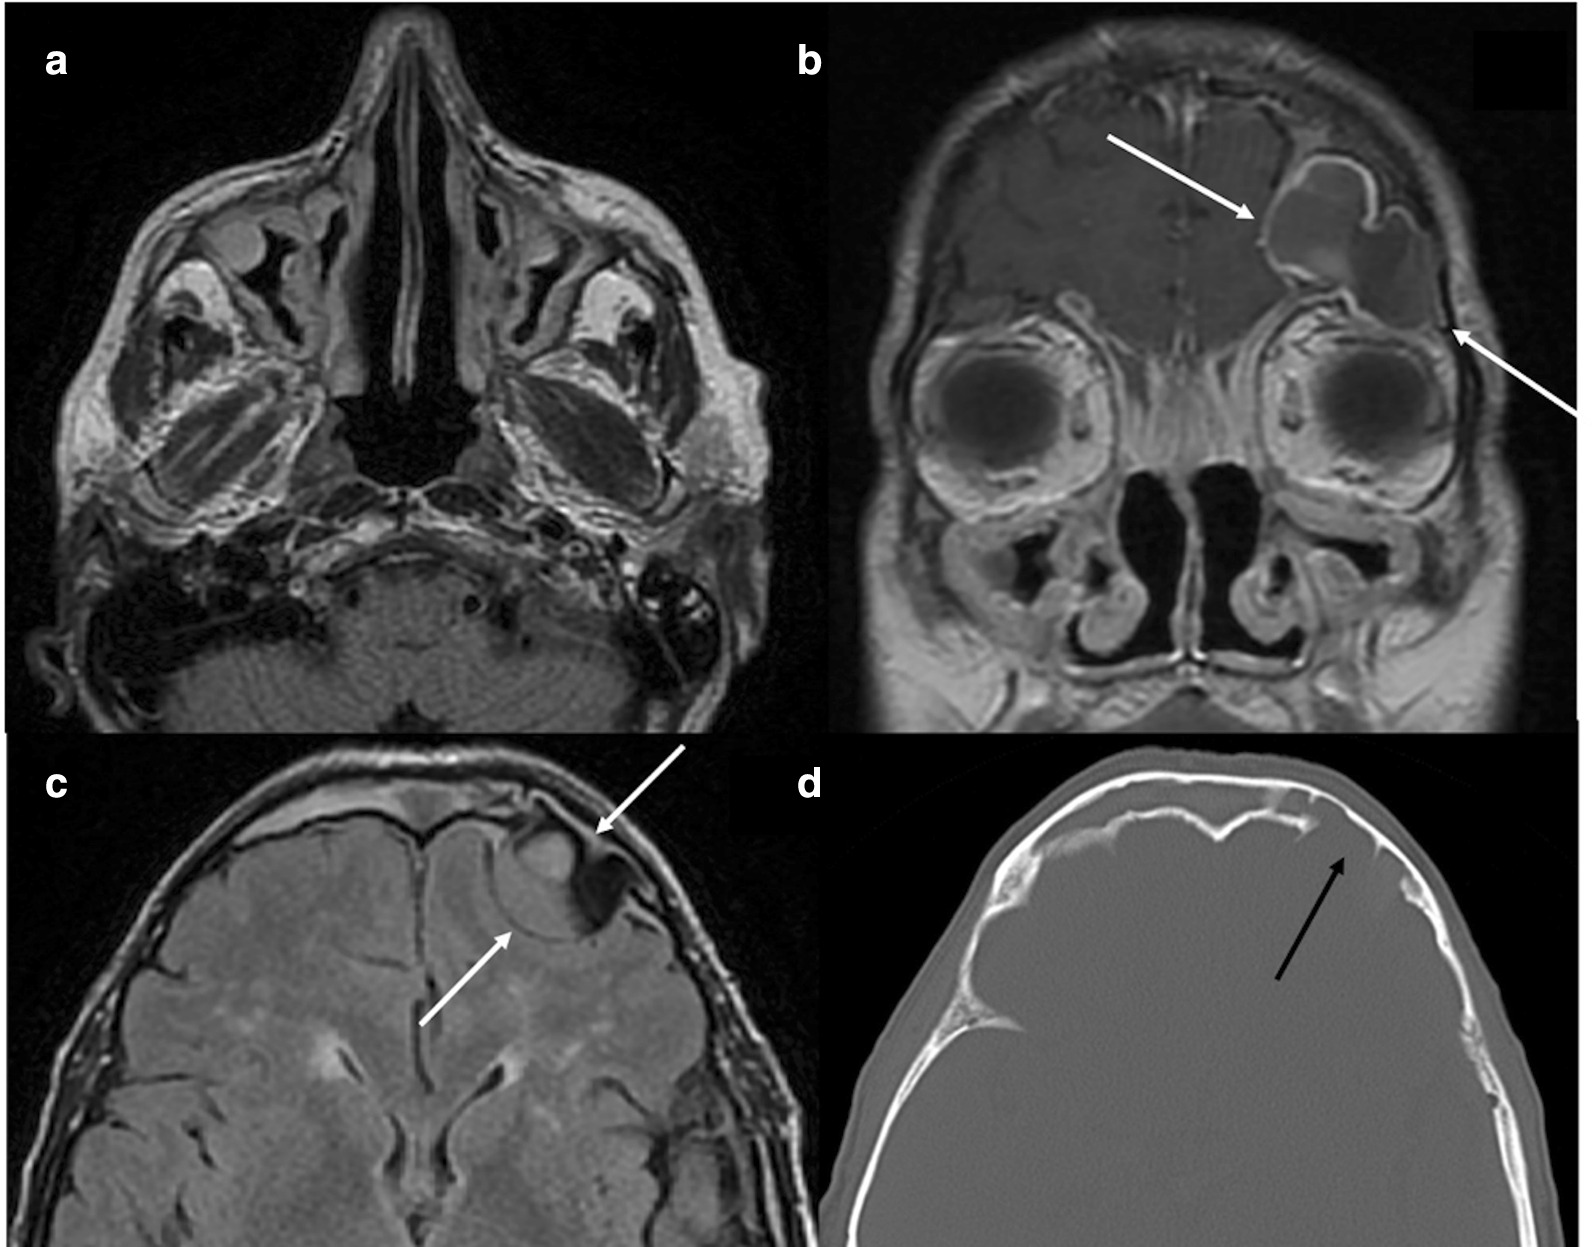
Axial T2-weighted MR image shows slightly hyperintense thickened mucosa in maxillary sinuses with concomitant retained secretions bilaterally (a). Preoperative coronal T1-weighted gadolinium enhanced MR scan. A multiloculated in homogeneously enhancing lesion protrudes from the left frontal sinus to the left frontal region, corresponding to a large mucocele (white arrows). Findings of superior and middle turbinectomy as well as bilateral maxillary antrostomy may be clearly depicted with slightly hyperintense thickened mucosa in both maxillary sinuses (b). Axial FLAIR MR sequence. A multiloculated lesion corresponding to a large mucocele, protrudes to the left frontal subarachnoid space, abutting the left frontal lobe (white arrows). The lesion shows internal areas of variable signal intensity, ranging from high to low (c). Brain CT in axial plane, bone window setting. The large mucocele causes an extensive erosion of the posterior wall of the left frontal sinus, protruding intracranially (black arrow) (d)
